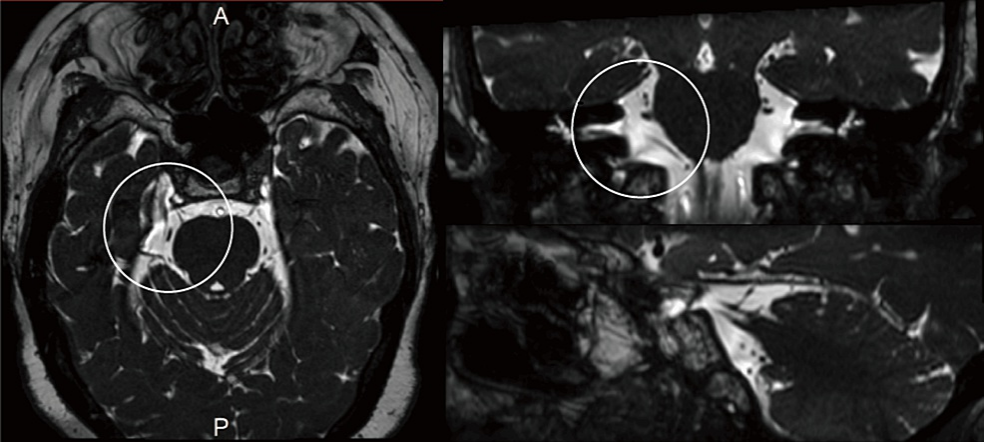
MRI 3D CISS image The image shows no vascular compression anywhere along the trigeminal nerve CISS: constructive interference in steady state; MRI: magnetic resonance imaging
